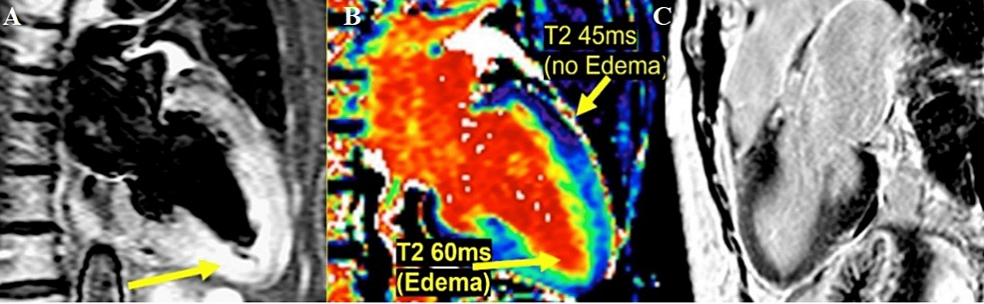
CMR examination of a 68-year-old female patient with Takotsubo cardiomyopathy CMR: Cardiac magnetic resonance Vertical long axis (VLA) views from left to right : A shows a T2-weighted STIR image. B shows T2 mapping image showing apical circumferential subepicardial oedema (yellow arrows). C shows late gadolinium enhancement image shows no area of hyperenhancements suggesting the absence of focal myocardial fibrosis. Image courtesy: Personal archive of Dr. Oana Popa. Patient consent was obtained.
